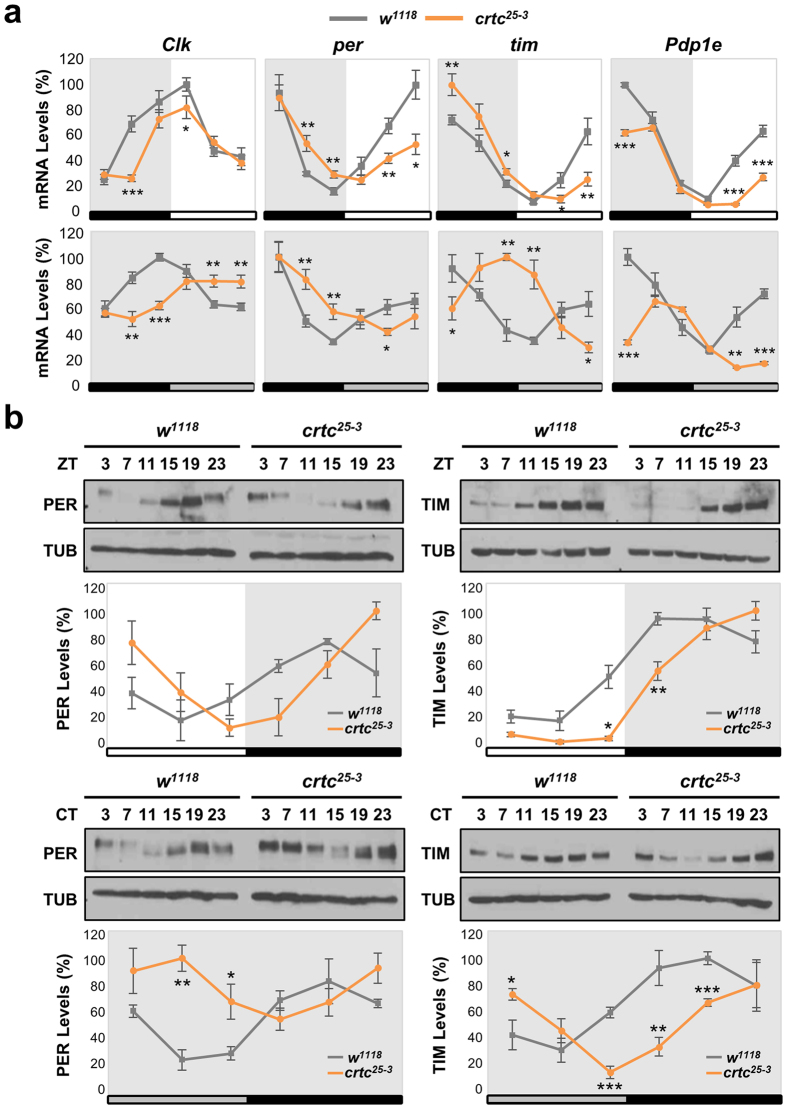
crtc* mutation phase-delays circadian gene expression in adult fly heads. (a) Circadian expression of clock mRNAs in adult fly heads of wild-type (gray lines) and *crtc* mutants (orange lines). Flies were collected at six different time-points in LD cycles (top) or during the transition from the first to the second DD cycle following LD entrainment (bottom) and total RNAs from heads were purified. Relative levels of *Clk*, *per*, *tim*, and *Pdp1* mRNAs were quantified by real-time RT-PCR. X-axis indicates zeitgeber time (ZT) in LD (top) or circadian time (CT) in DD (bottom). Y-axis indicates relative expression levels (%) at each time-point, calculated by normalizing to the peak value (set as 100). White/black bars, LD cycles; gray/black bars, DD cycles. Data represent the average of three independent experiments. P* < 0.05, *P* < 0.01 and P* < 0.001 as determined by Student’s *t*-test. Error bars indicate SEM. (b) Circadian expression of PER and TIM proteins in adult fly heads of wild-type (gray lines) and *crtc* mutants (orange lines). Head extracts were prepared from flies harvested during LD (top) or the first DD (bottom) cycle and immunoblotted with anti-PER, anti-TIM and anti-TUBULIN (TUB, loading control) antibodies under the same experimental conditions. Images were cropped from full-length blots shown in Supplementary Fig. 12. Protein band intensities in each lane were quantified using ImageJ software and normalized to that of TUB protein. Y-axis indicates the relative expression levels (%) of PER and TIM proteins, calculated by normalizing to the peak value (set as 100). White/black bars, LD cycles; gray/black bars, DD cycles. Data represent the average of three independent experiments. P* < 0.05, *P* < 0.01 and P* < 0.001 as determined by Student’s *t*-test. Error bars indicate SEM.
